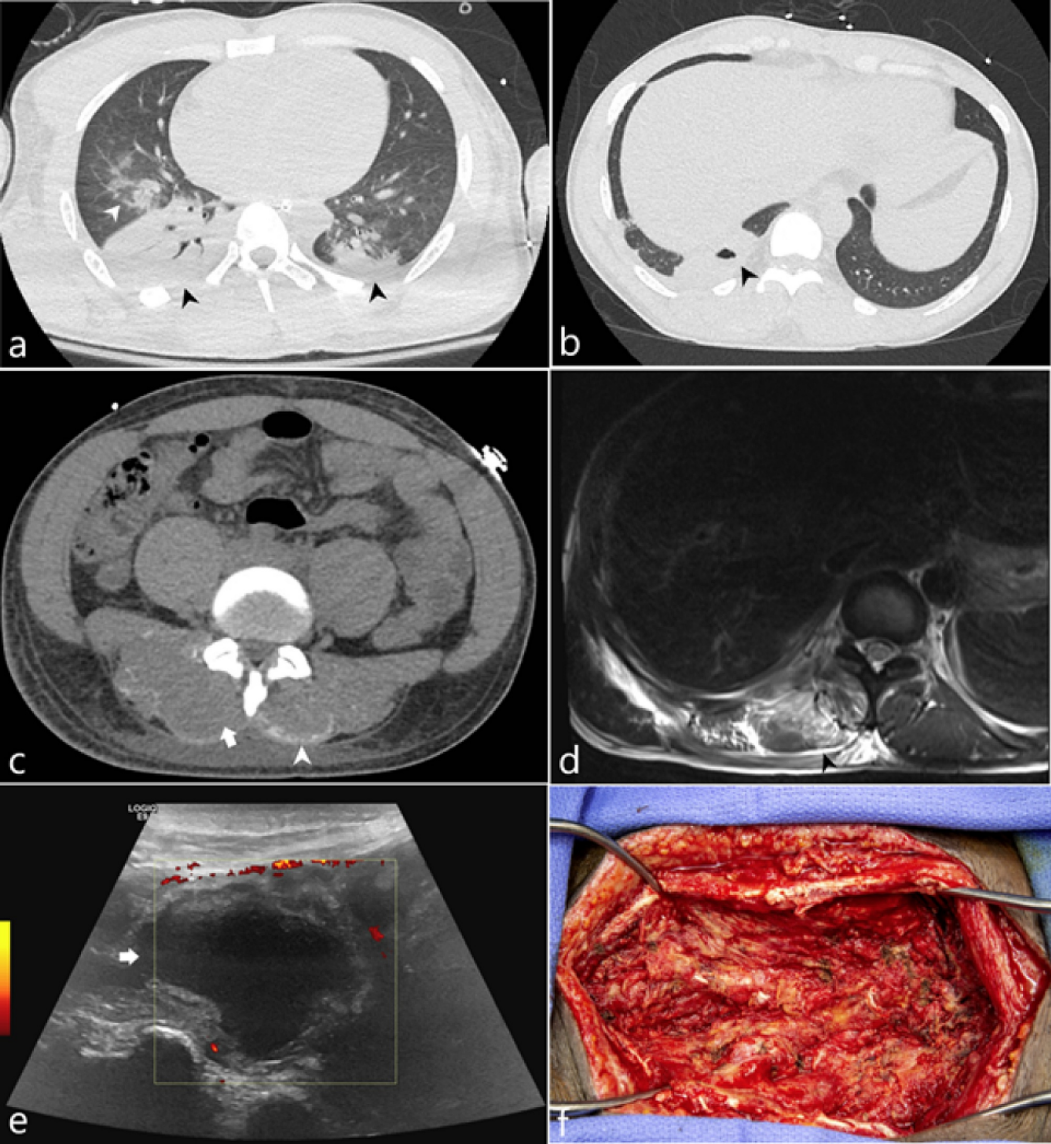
33-year-old soldier with sickle cell trait was admitted due to rhabdomyolysis complicated by COVID-19 positivity (a) Axial CT image of the lung bases acquired on hospital day 3 demonstrates consolidation (black arrowheads) and ground glass opacities (white arrowhead). (b) Axial CT image of the lung base acquired on hospital day 16 demonstrates a cavitation (black arrowhead). (c) Axial CT image of the lumbar spine at the level of the L1 vertebral body on hospital day 16 demonstrates bilateral curvilinear regions of hyperattenuation along the posterior margin of the paraspinal muscles (white arrowhead) as well as a hypoattenuating appearance of the paraspinal muscles. (d) MRI of the lower thoracic spine at the T11 level demonstrates T2 hyperintense signal (black arrowhead) of the paraspinal muscles representing edema. (e) Sonographic evaluation of the paraspinal muscle approximately 43 days from initial admission demonstrates an anechoic fluid collection (white arrow) with a thickened peripheral rim. (f) Operative image of the paraspinal soft tissues status post evacuation.
